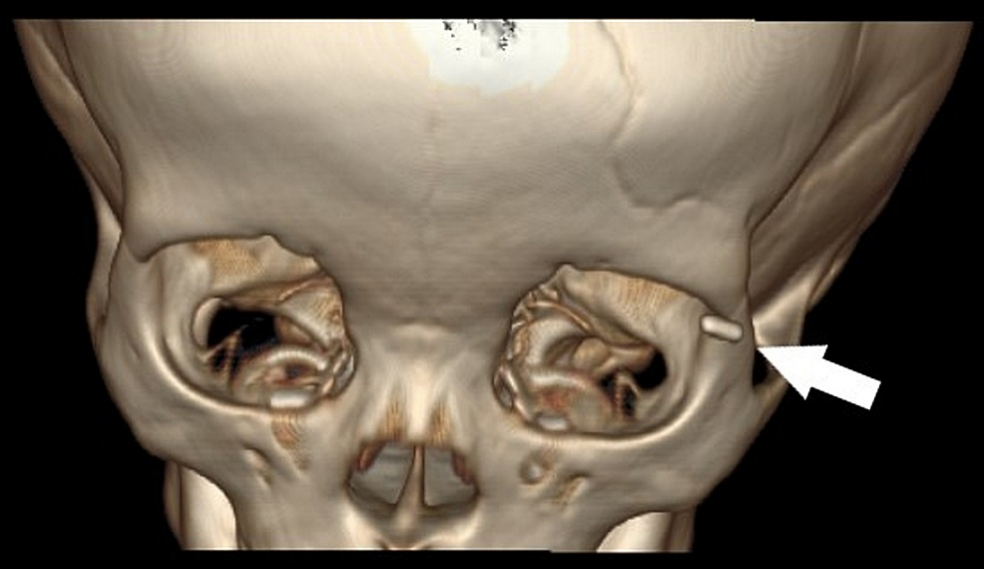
CT 3D reconstruction showing the exact location of the foreign body (white arrow)
